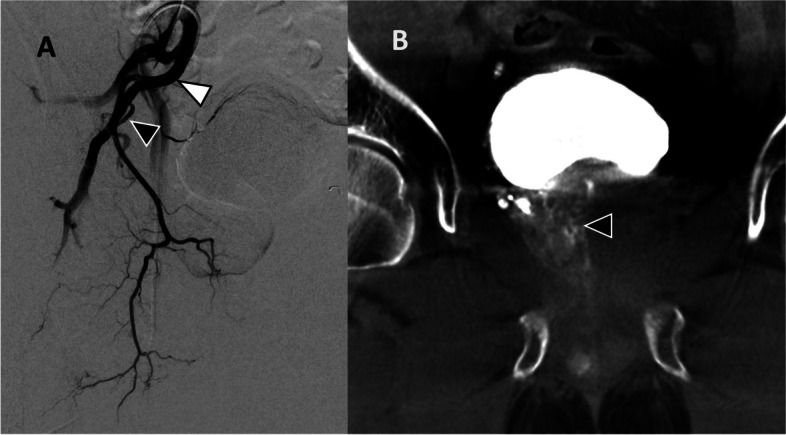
A DSA of the right internal iliac artery (white arrowhead) showed a variant right prostatic artery arising from the right inferior gluteal artery (black arrowhead). B CBCT after cannulation of the variant right prostate artery confirmed perfusion of the right lobe (black arrowhead). Prostate was not extremely enlarged (73 mL) and the prostate blush could be inconclusive without CBCT confirmation
